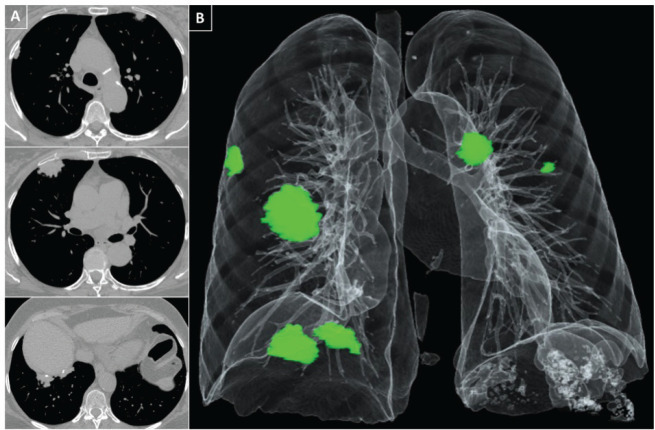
(A) Axial computed tomography (CT) slices in a craniocaudal sequence
